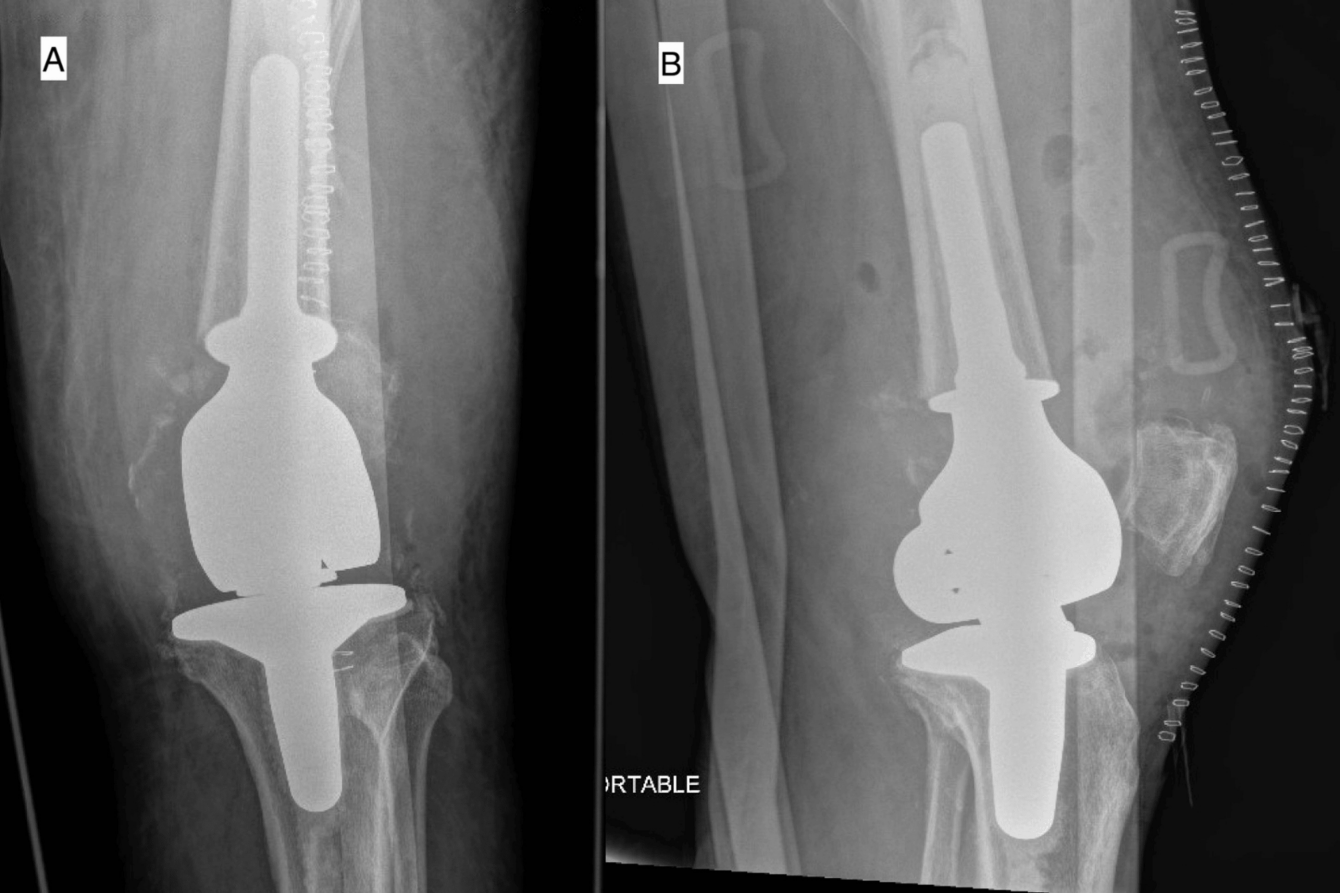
Distal femur replacement postoperative (A) anteroposterior and (B) lateral radiographs.
